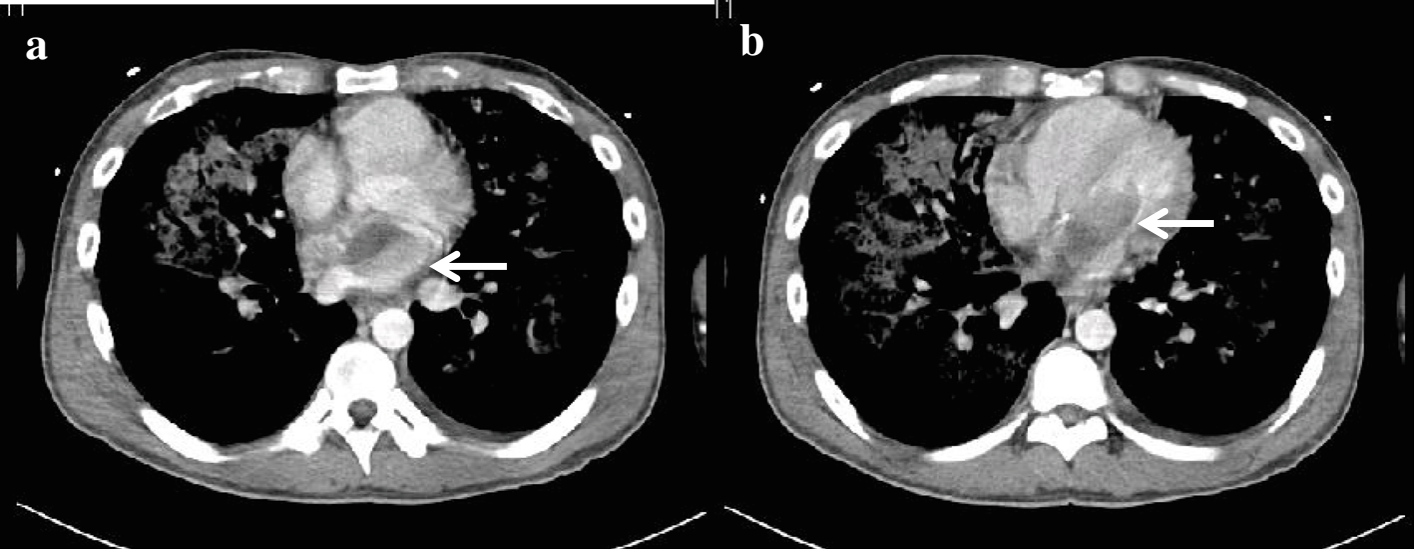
Preoperative enhanced computed tomography scan. It revealed a large tumor in the left atrium (arrow) (a), incarcerating into the mitral annulus (arrow) (b)
